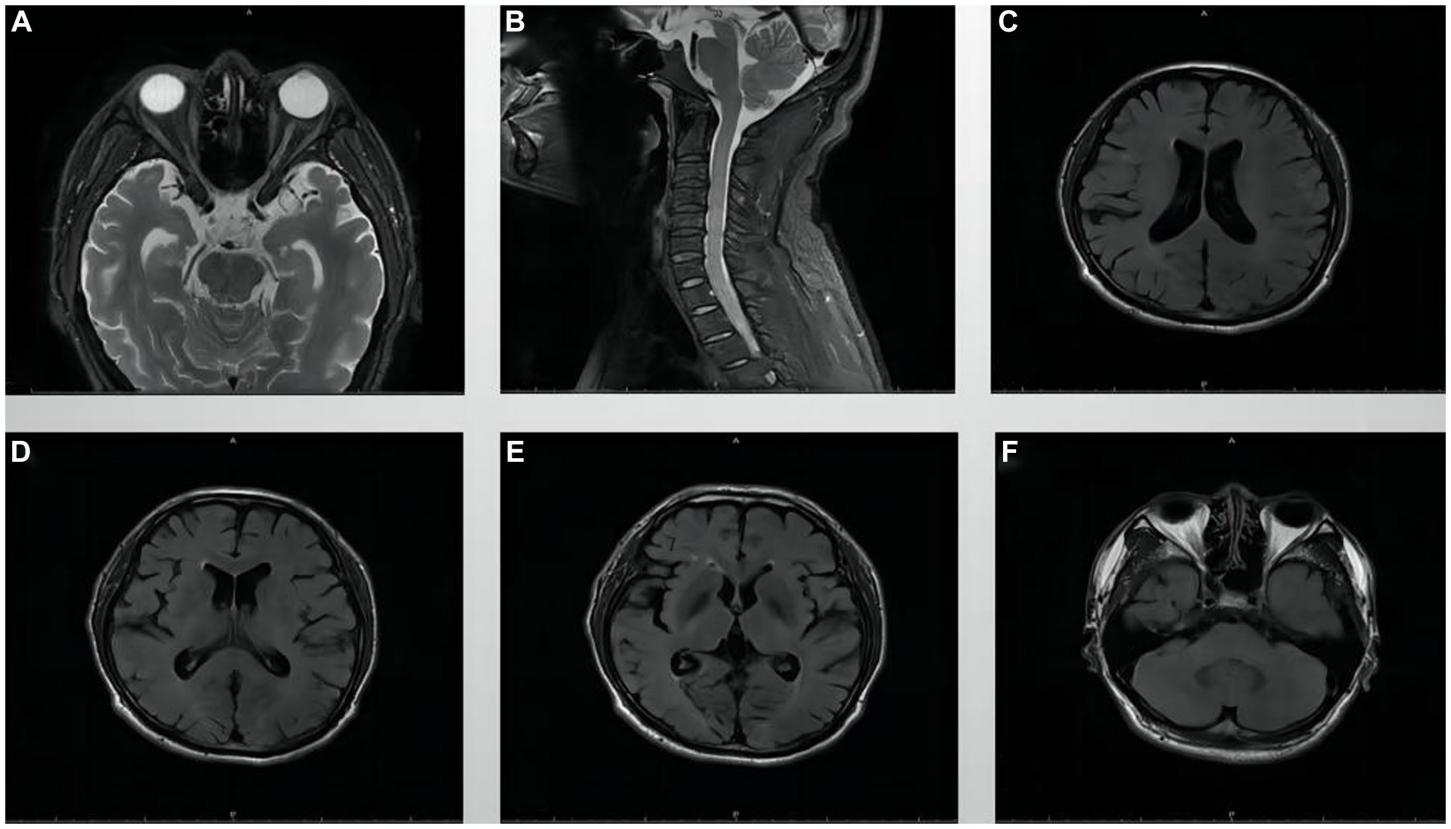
MRI images of the orbit, cervical spine, and brain. (A) Orbital MRI, (B) Cervical spine MRI, and (C–F) Brain MRI.
